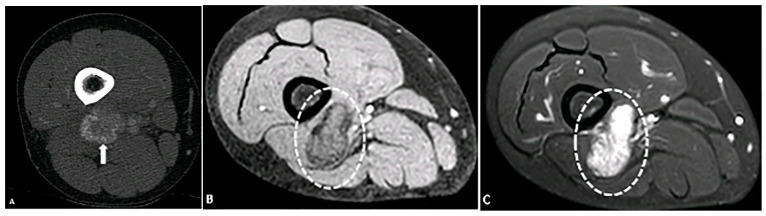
A 33-year-old female with a mass in the posterior distal aspect of the right leg. (A) Axial contrast-enhanced CT shows a mass with peripheral calcification (arrow). (B) Axial T1-weighted fat-suppressed image shows a mass (circle) with a peripheral hypointense line indicative of calcification and central hyperintensity (circle) on a T2-SPAIR fat-suppressed image (C). Findings are most consistent with myositis ossificans in the setting of recent trauma. The image was obtained from the University of Washington Medical Imaging database with the required permissions.
